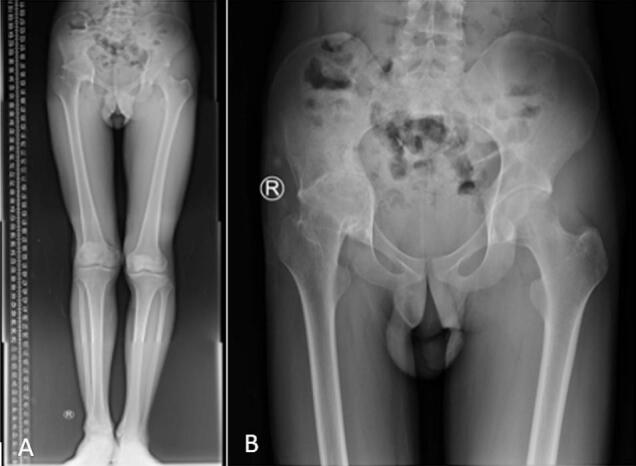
A plain radiograph of scanogram (A) and pelvic AP (B) shows ankylosing of the right hip joint.
